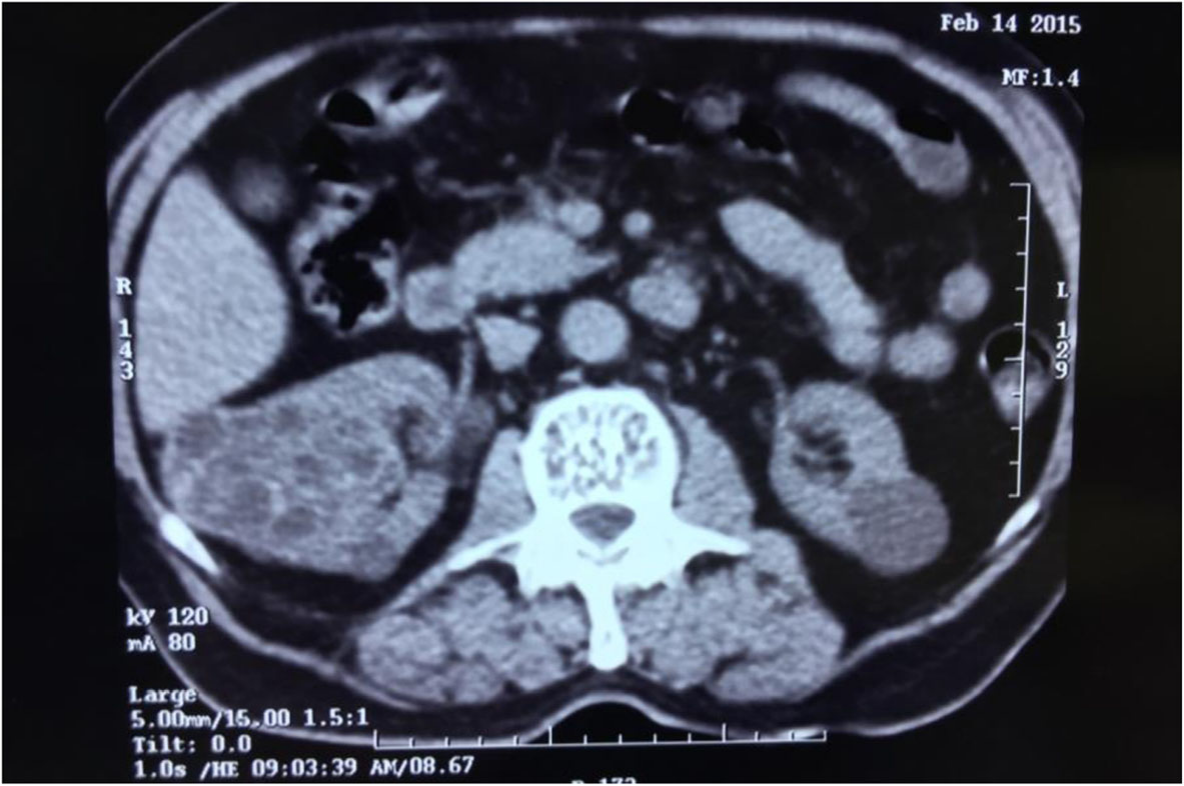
CT-scan of the patient on initial admission at our center showing multilocular cyst at right kidney
